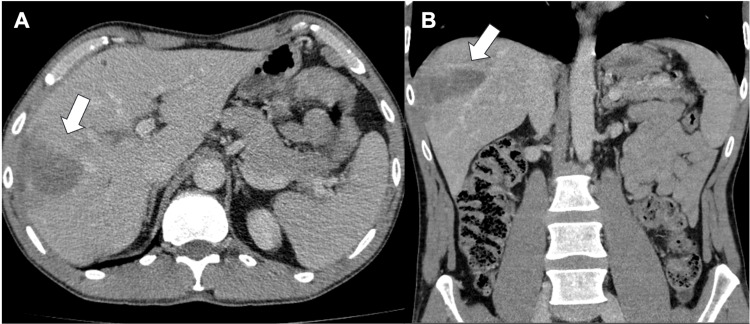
Abdominal CT scan showing the liver after treatment A) Axial abdominal computed tomography (CT) image showing a poorly defined, heterogeneous, hypodense area in the right mid-lobe of the liver (arrow); B) Coronal CT reconstruction showing the extent of the lesion, measuring approximately 3×6×6 cm, and appearing less organized compared to the previous examination.
